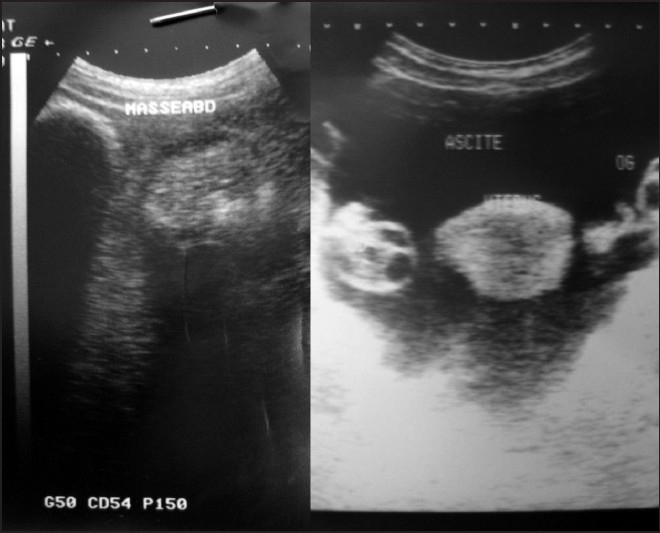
Ultrasonography showing a heterogeneous image in the left upper quadrant and mild ascites
